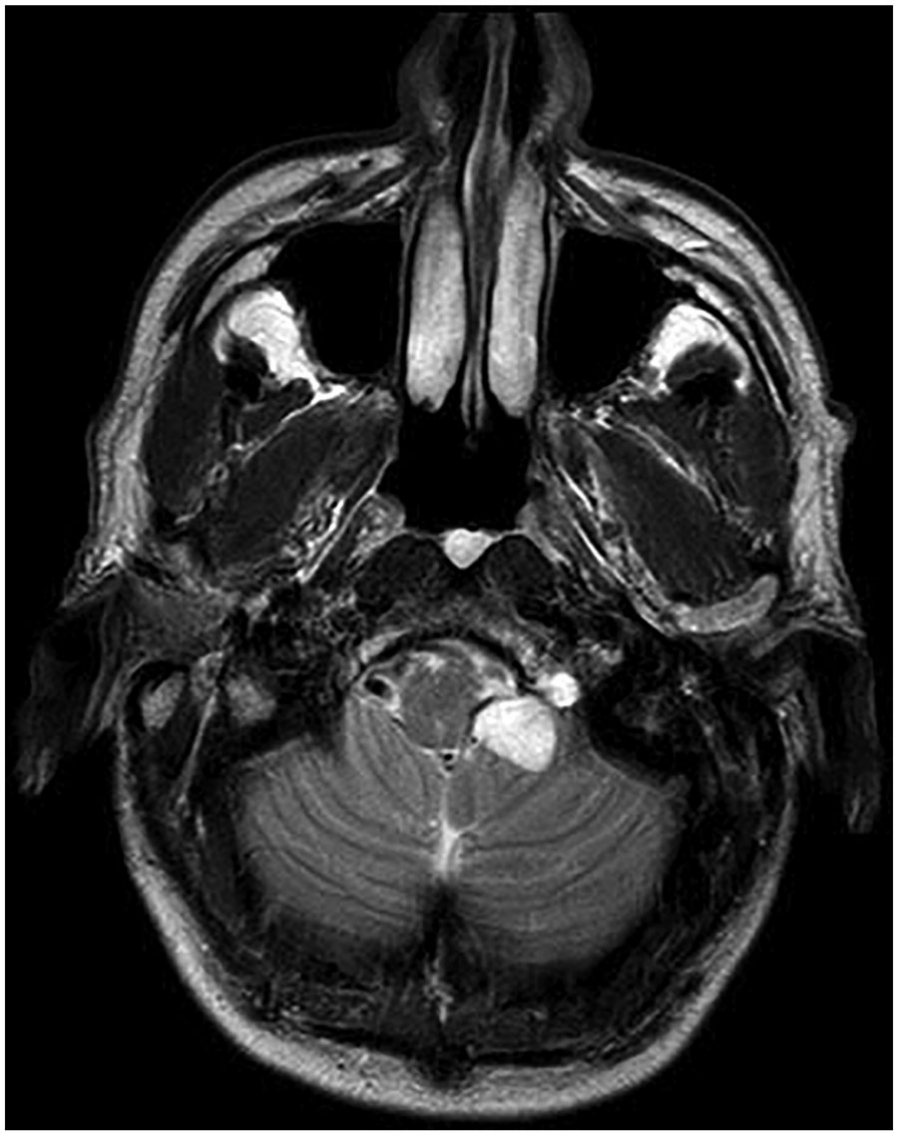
Axial T2 weighted magnetic resonance image (MRI) demonstrating lesion in the left hypoglossal foramen being T2 hyperintense with no gadolinium contrast enhancement.
